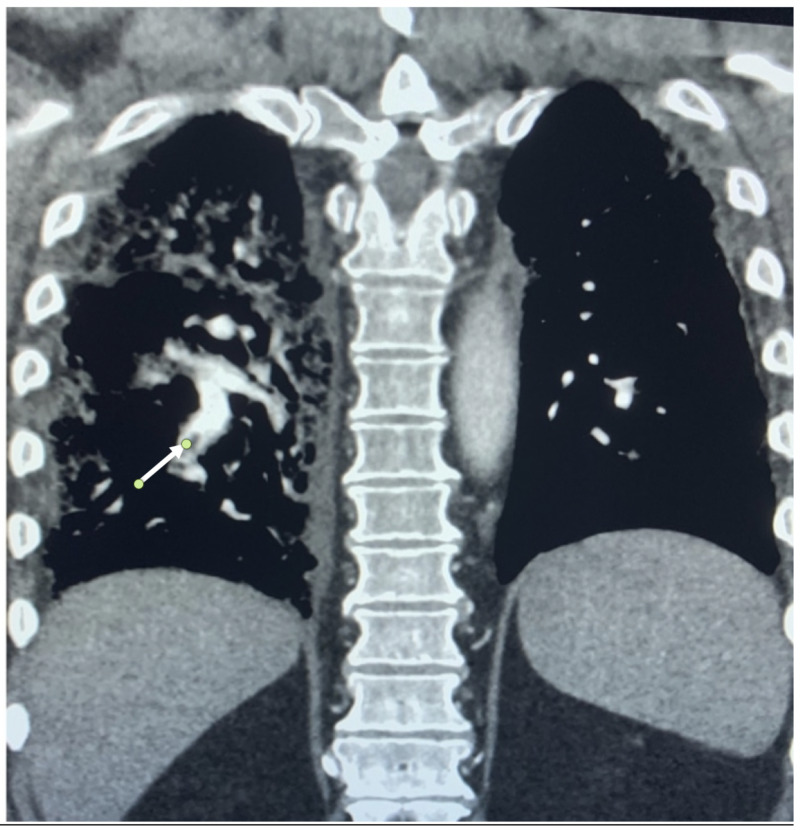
Coronal chest CT showing filling defect (white arrow) within the right lower lobe segmental pulmonary artery CT: computed tomography
